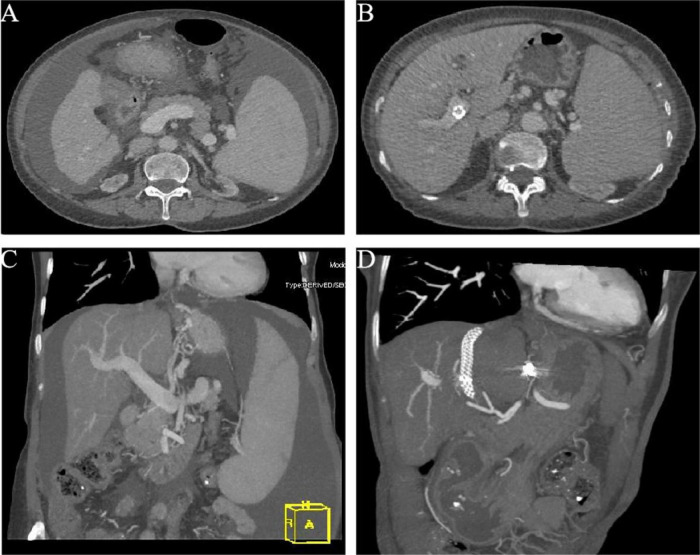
Abdominal enhanced computed tomography before TIPS (A) and after TIPS (B) in the case. Three-dimensional computed tomography (CT) images before TIPS (C) and after TIPS (D) demonstrate significant resolution of ascites post-procedure. TIPS, transjugular intrahepatic portosystemic shunt.
